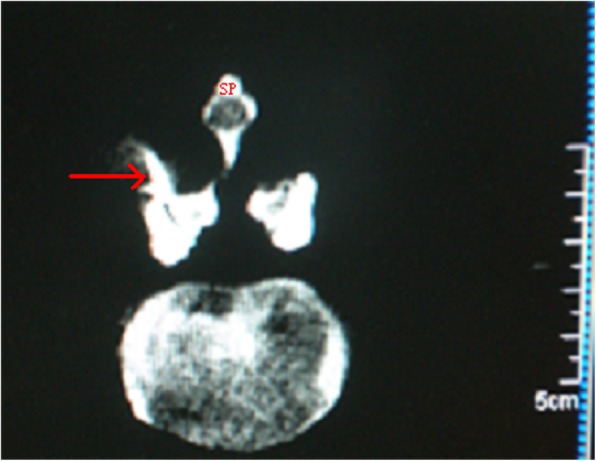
The needle tip verified under the CT monitoring. SP: Spinous process; arrow: needle
